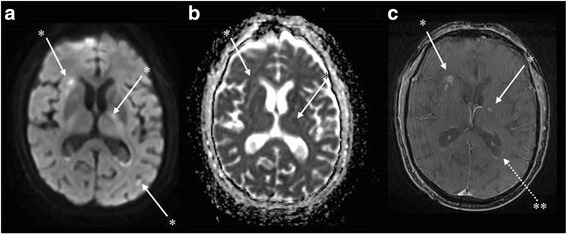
Brain magnetic resonance imaging (day 6) showing possible cerebral vasculitis in multiple axial sequences. *Punctiform hyperintensities in diffusion-weighted imaging (a) in several vascular territories with cortical and sub-cortical distribution. These lesions appear with a normal or decreased axial apparent diffusion coefficient value (b) and some are enhanced after gadolinium injection in T1 sequences suggesting a blood-brain barrier disruption (c) and consistent with semi-recent ischemic lesions. Ependidymal enhancement in T1 with gadolinium enhancement sequences, sign of ventriculitis (c)
